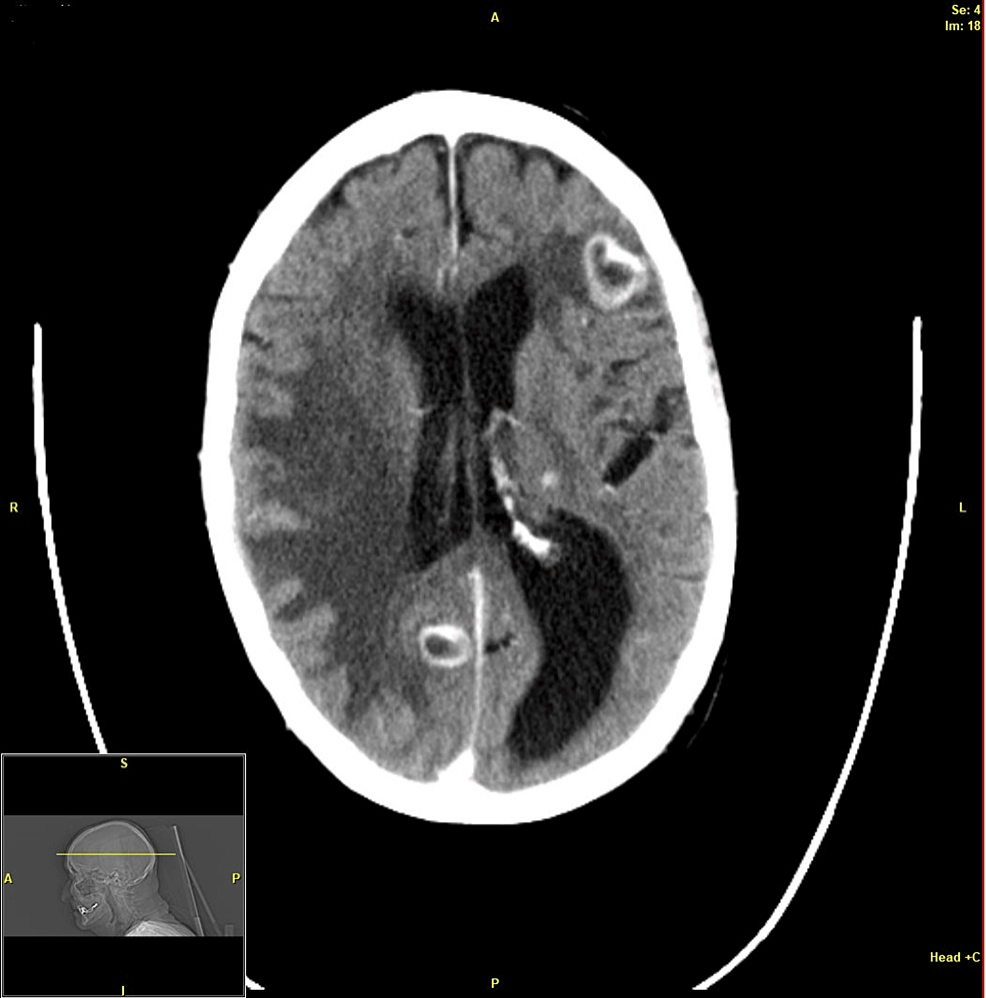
CT of the head with contrast enhancement showing ring-enhancing subcortical lesions.
